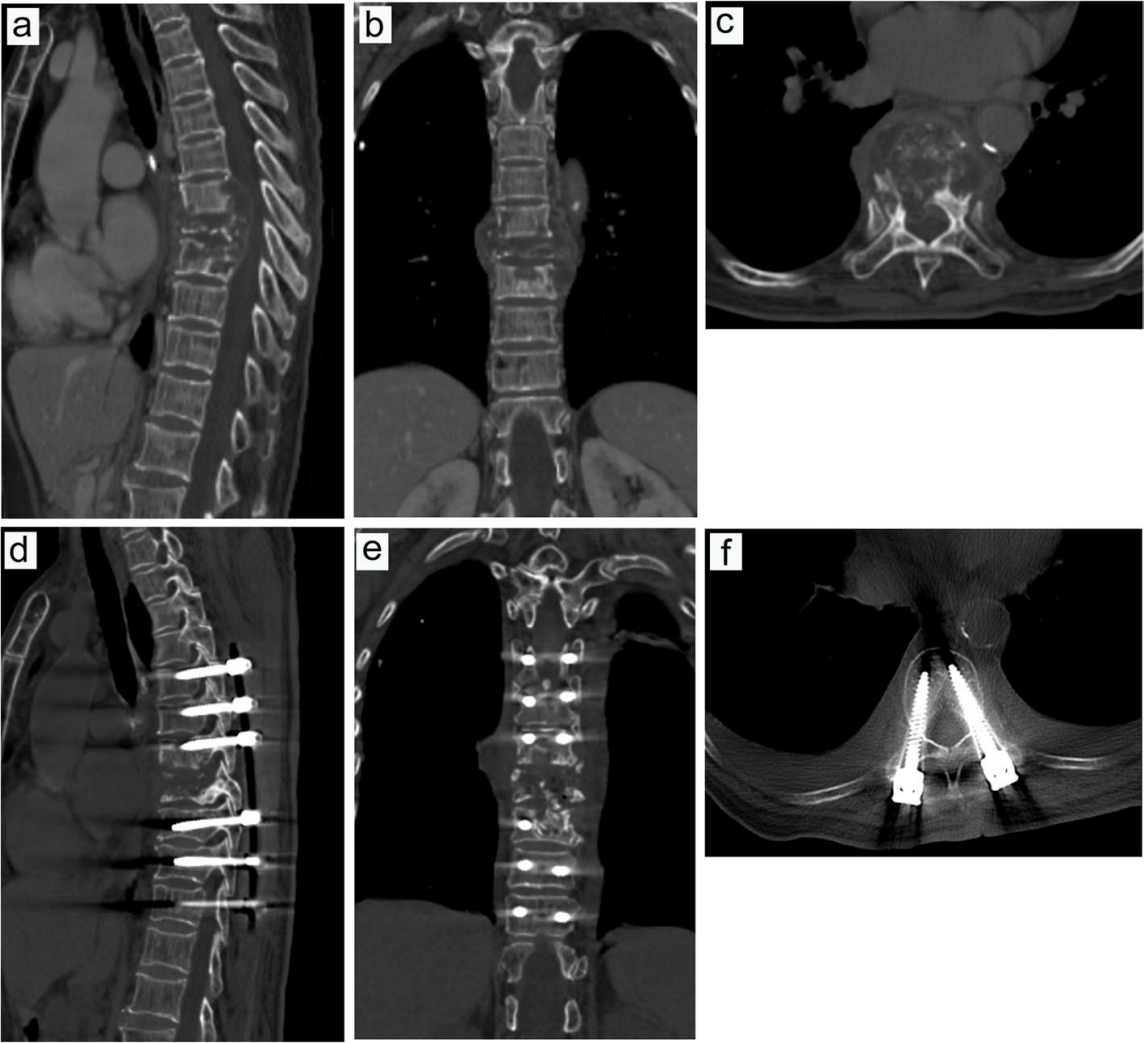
Preoperative and postoperative CT examinations in one patient with intraoperative blood transfusion. a, Preoperative CT examination in the sagittal position. b, Preoperative CT examination in the coronal position. c, Preoperative CT examination in cross-section. d, Postoperative CT examination in the sagittal position. e, Postoperative CT examination in the coronal position. f, Postoperative CT examination in cross-section
